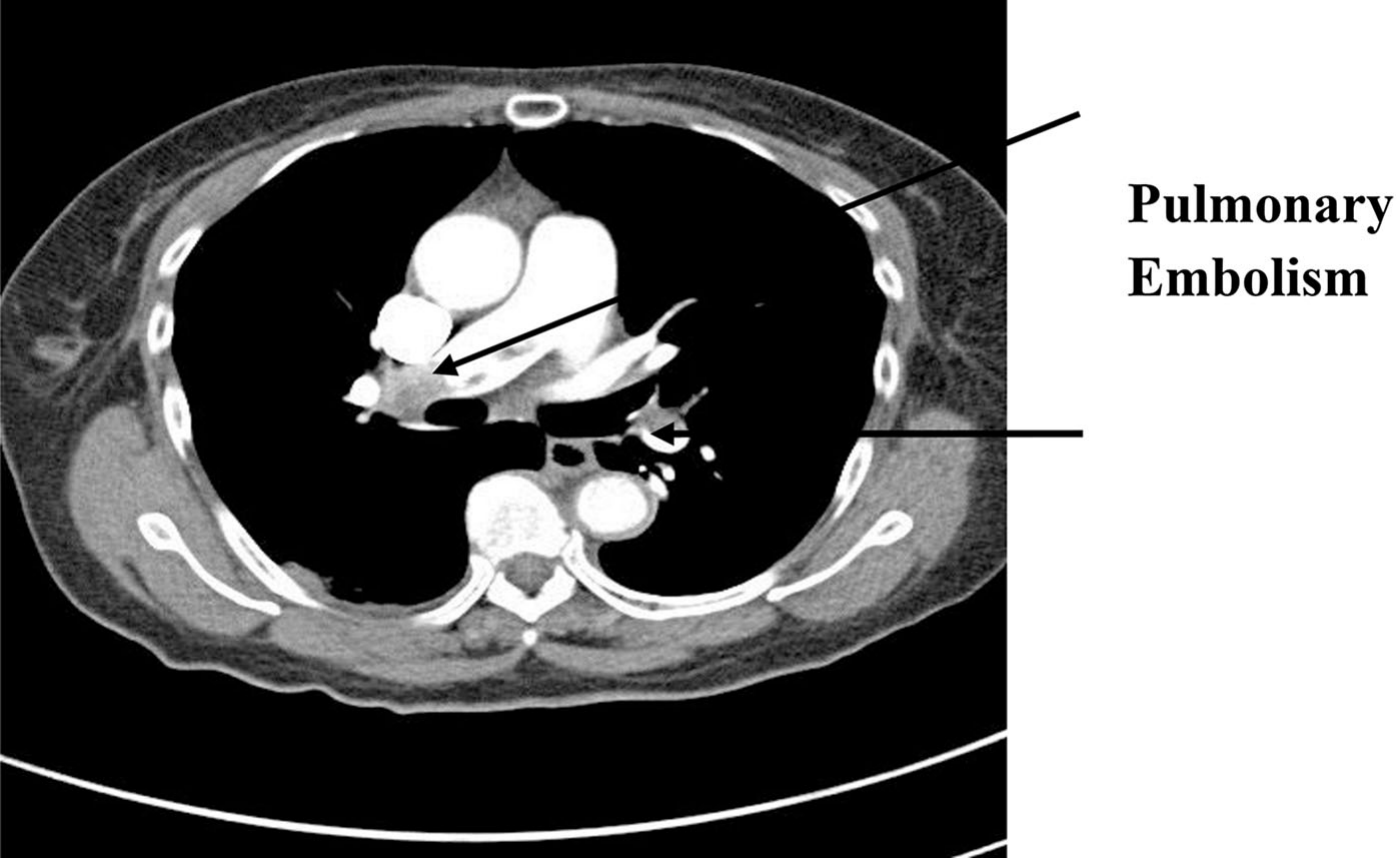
CT angiogram showing bilateral pulmonary emboli.
